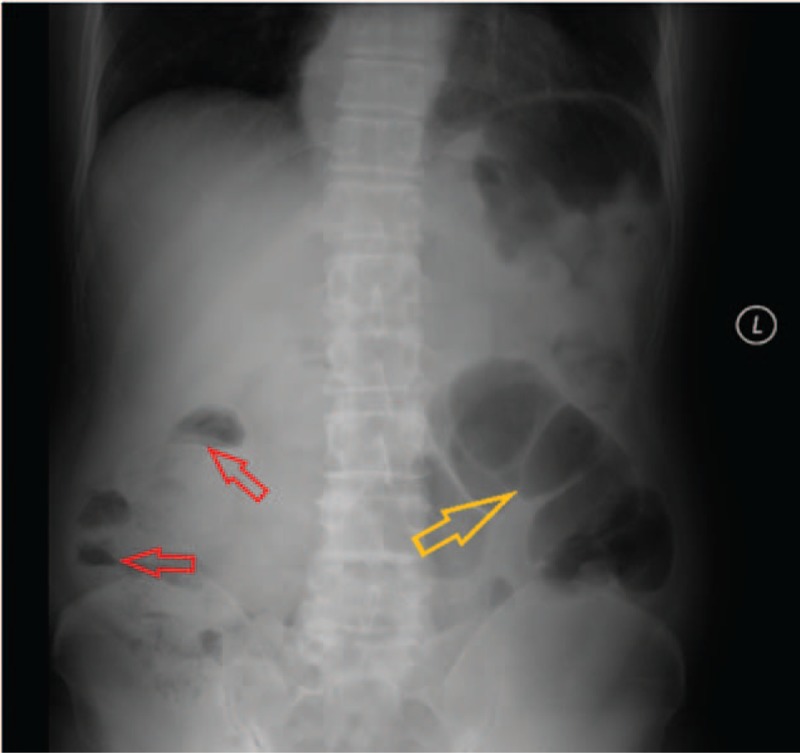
Abdominal radiography revealed air-fluid levels (red arrows) and massive gas accumulation in the dilated intestinal canal (yellow arrow).
